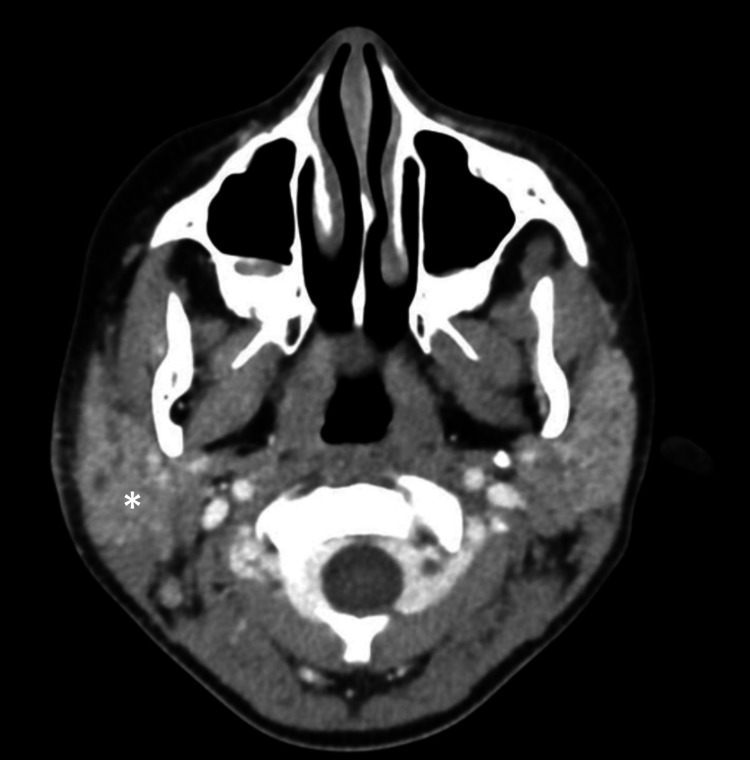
CT scan with contrast of the head and neck showing right parotitis with adjacent soft tissue edema and reactive lymphadenopathy (*)
